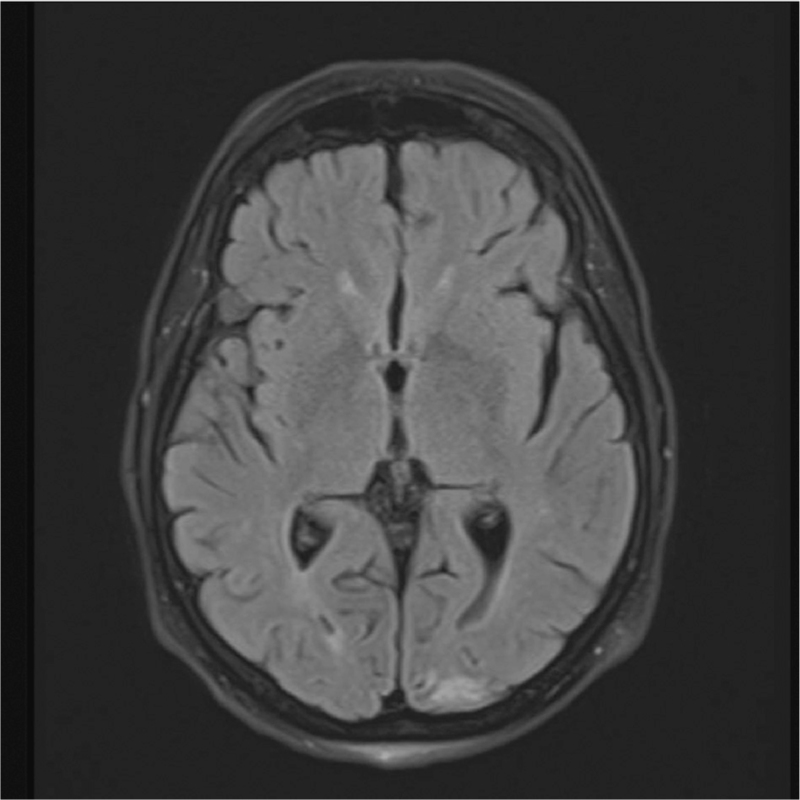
Axial FLAIR MRI showing diffusion restriction in left occipital lobe.
